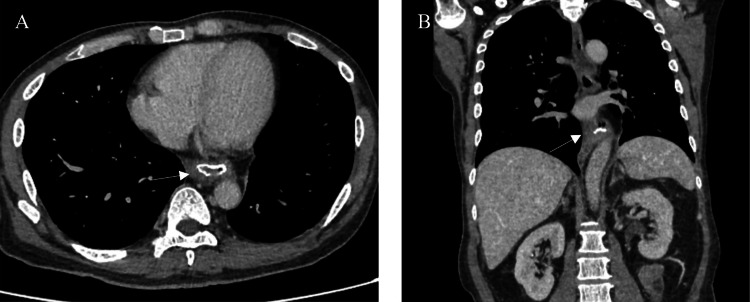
Contrast-enhanced CT of the thorax and abdomen in axial (A) and coronal (B) view showing enhancing thickening at esophagojejunostomy anastomotic site suggestive of possible local recurrence
